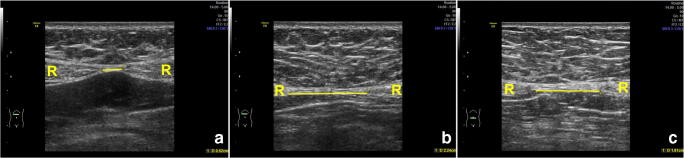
Ultrasound images of the inter-rectus distance at 5 cm above (a), 2 cm above (b), and 2 cm below (c) the umbilicus. R: Rectus abdominis. Yellow line: Measurement between the two rectus abdominis muscles at the low edge of the connection
